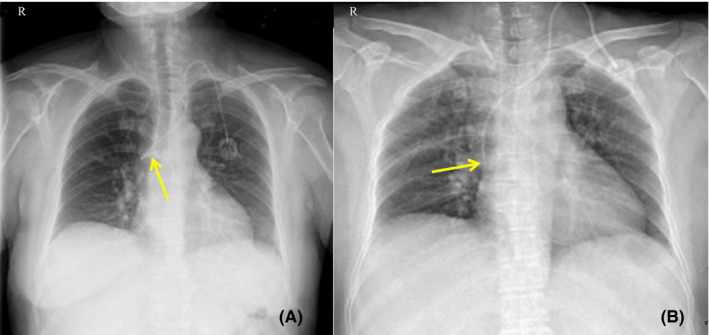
X‐ray radiography findings in Case 1. A, The first postoperative X‐ray radiographic examination showed that the catheter tip had entered AV. B, A second X‐ray examination showed that the catheter tip was correctly repositioned at the level of the 7th thoracic vertebra
